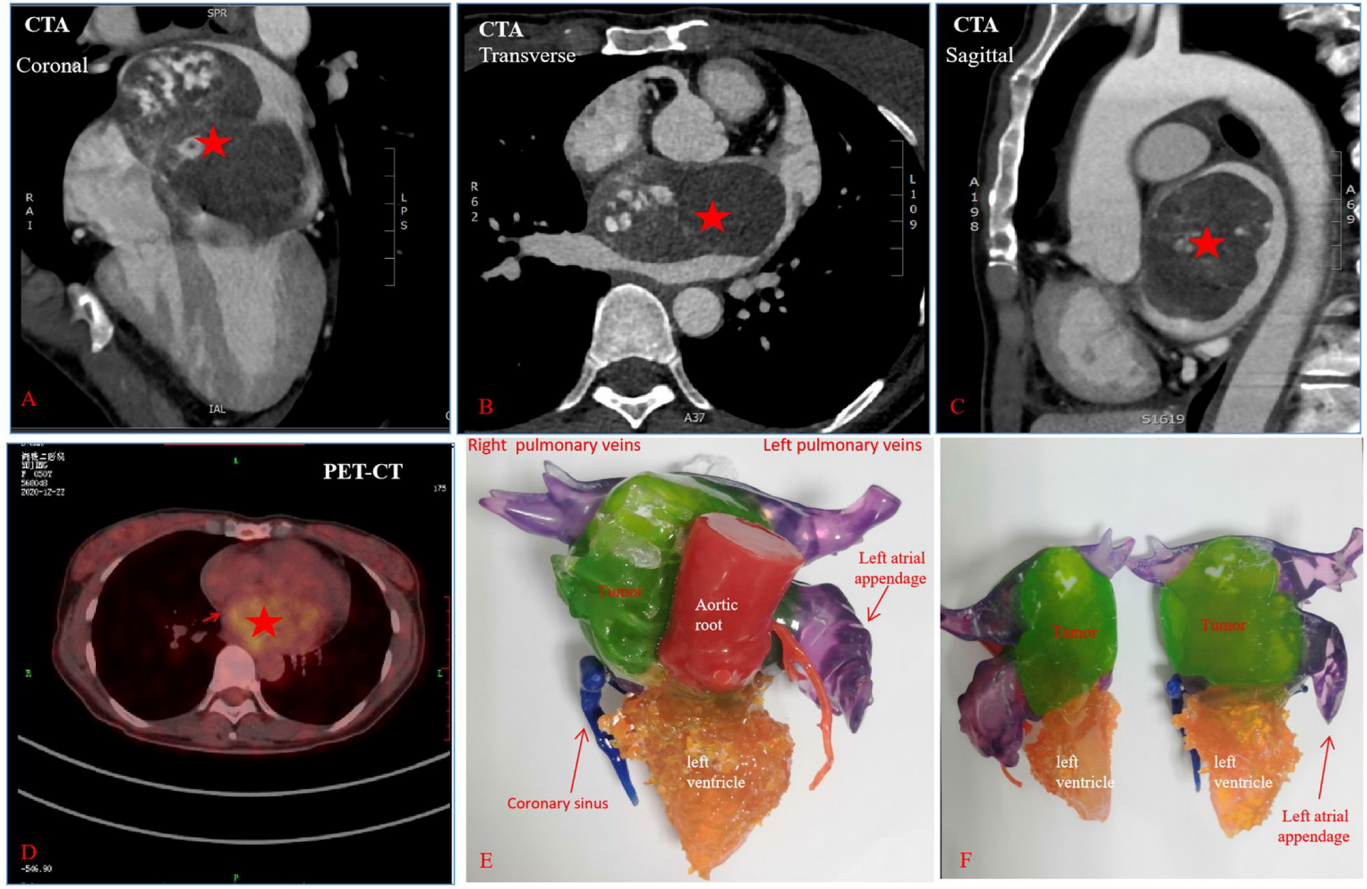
Pre-operative images showing left giant atrial tumor and adjacent structures. (A–C) Indicate images from CTA, (D) indicates image from PET-CT, (E,F) indicate images from 3D printing model. Red asterisks indicate left atrial tumor.
